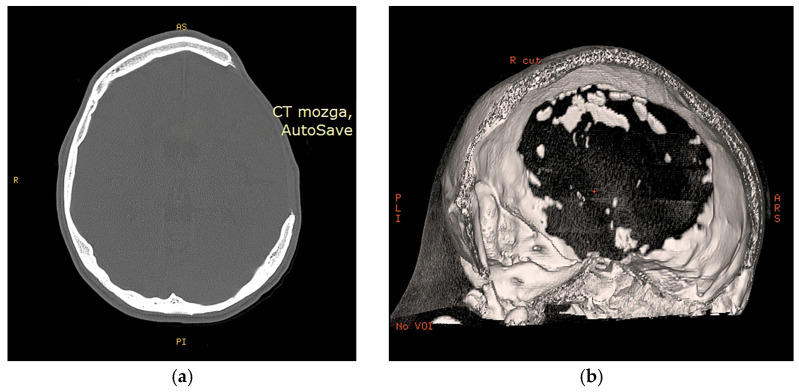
Second case CT scan: (a) transverse slice, and (b) CT-based 3D reconstruction.
